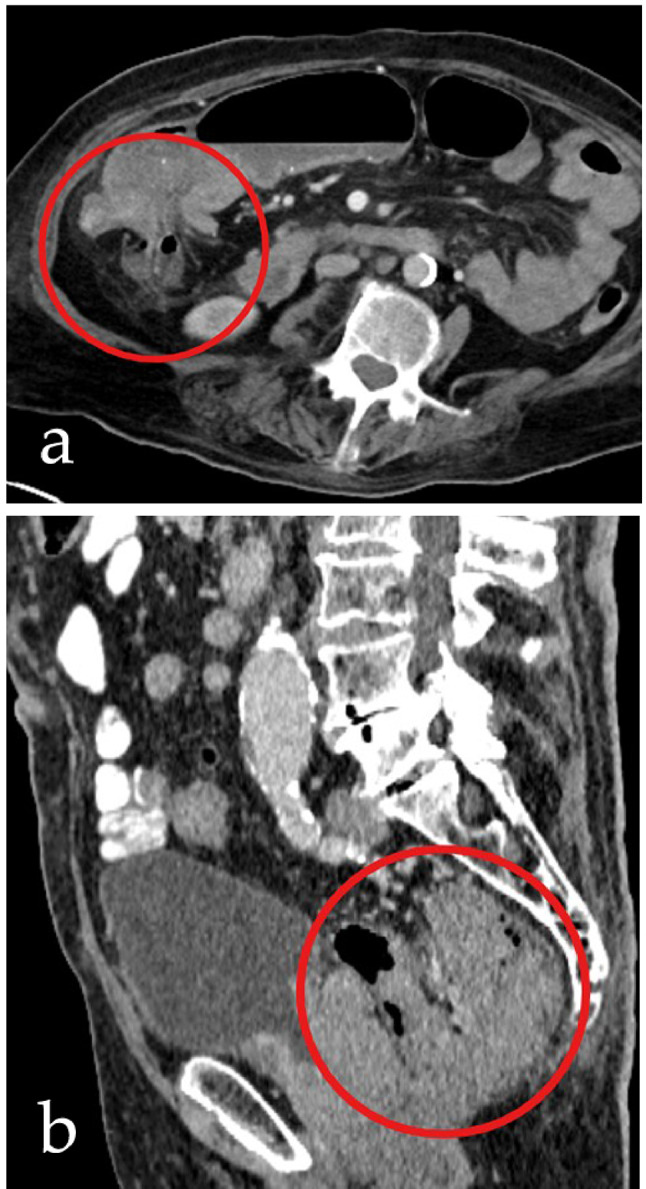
Contrast-enhanced CT images demonstrating adult intussusceptions secondary to malignant lead points. In panel (a), an axial image reveals a large, heterogeneous soft-tissue mass in the cecum (red circle) confirmed as an adenocarcinoma. In panel (b), a sagittal reconstruction shows a malignant mass in the rectum (red circle) acting as the lead point for the intussusception
